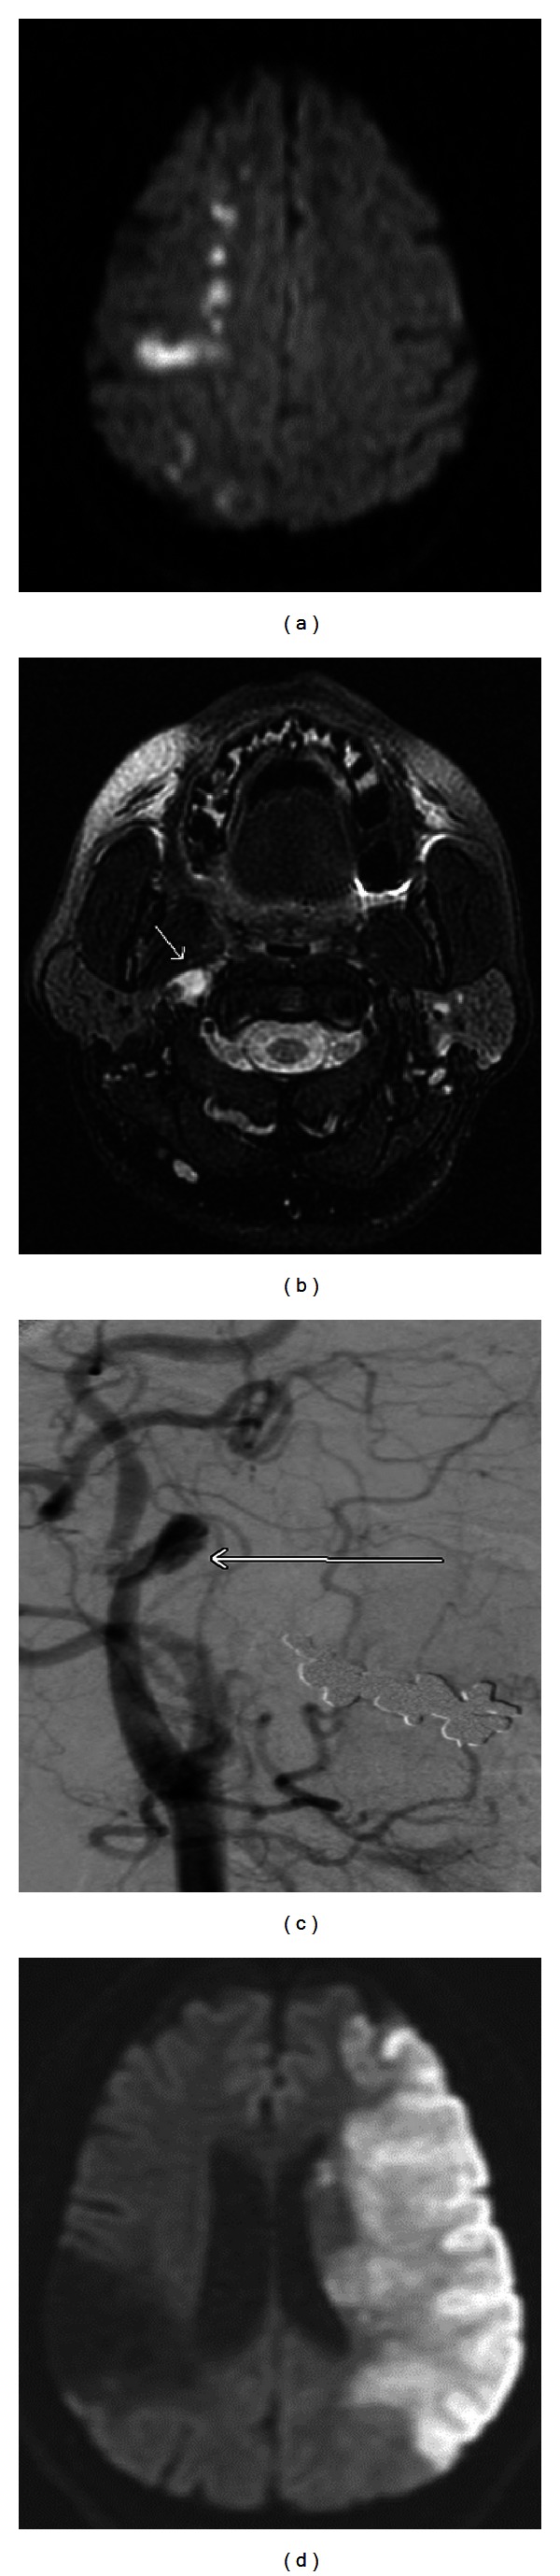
((a)–(d)) 39-year-old female presenting with acute onset of a right-sided headache associated with left hemiparesthesia and hemiparesis following sexual intercourse. (a) Initial brain MRI axial DWI sequence demonstrates abnormal restricted diffusion in a watershed distribution of the right cerebral hemisphere consistent with an acute stroke. (b) Axial T1-weighted fat-saturated sequence of the neck vessels demonstrates a hyperintense signal abnormality within the subintimal space of the right internal carotid artery (arrow) consistent with a dissection. (c) Conventional angiogram of the right internal carotid artery demonstrates a 360° loop with an associated fusiform aneurysm filled with thrombus (arrow). (d) Patient presented with acute onset of right hemiplegia and global aphasia 8 years after her first stroke. Brain MRI axial DWI sequence demonstrates a large region of abnormal restricted diffusion in the left cerebral hemisphere consistent with an acute stroke in the left middle cerebral artery distribution. There is encephalomalacia of the right parietal lobe in the region of her prior stroke.
